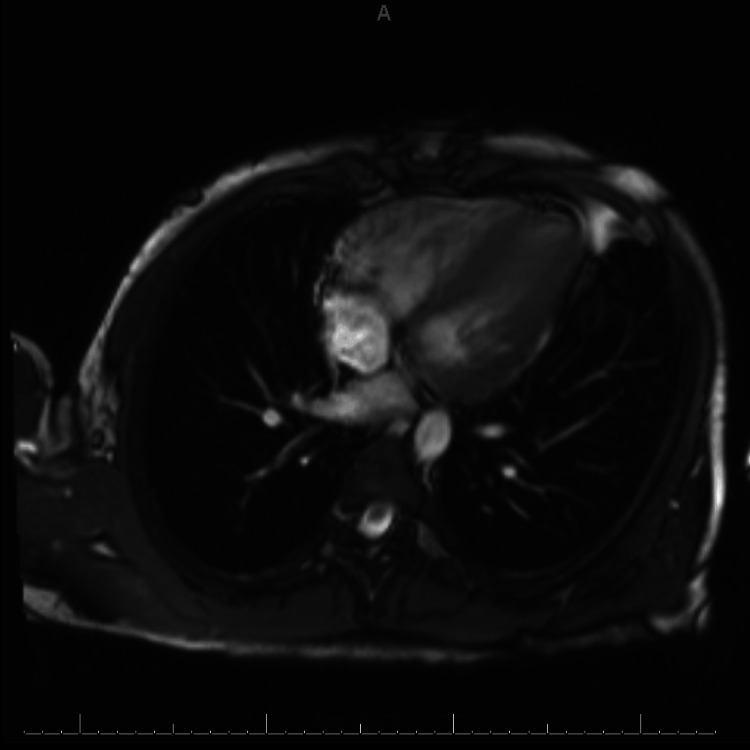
Cardiac magnetic resonance imaging (MRI) demonstrating normal left and right ventricular size and function with an ejection fraction (EF) of 61% and 65%, respectively. A small area of scar is visible in the apical anterior wall, which may be the nidus for the patient's dysrhythmia and arrest
